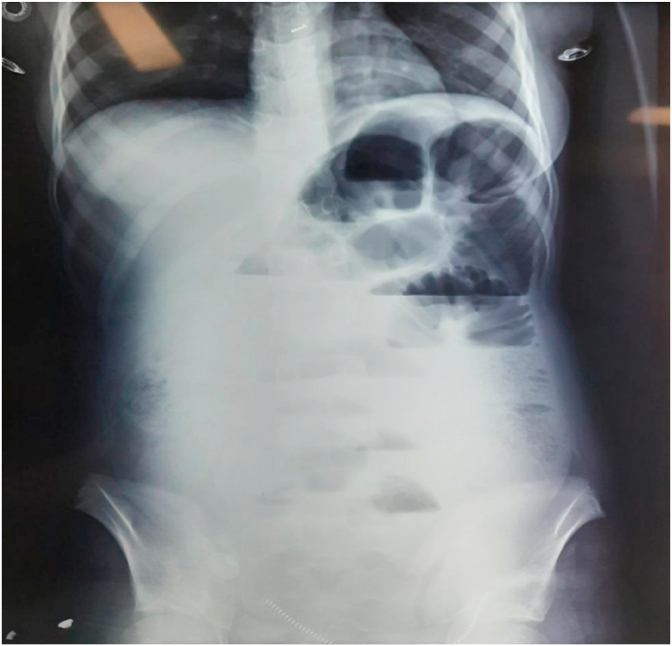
Plane X-ray showing small bowel obstruction.
